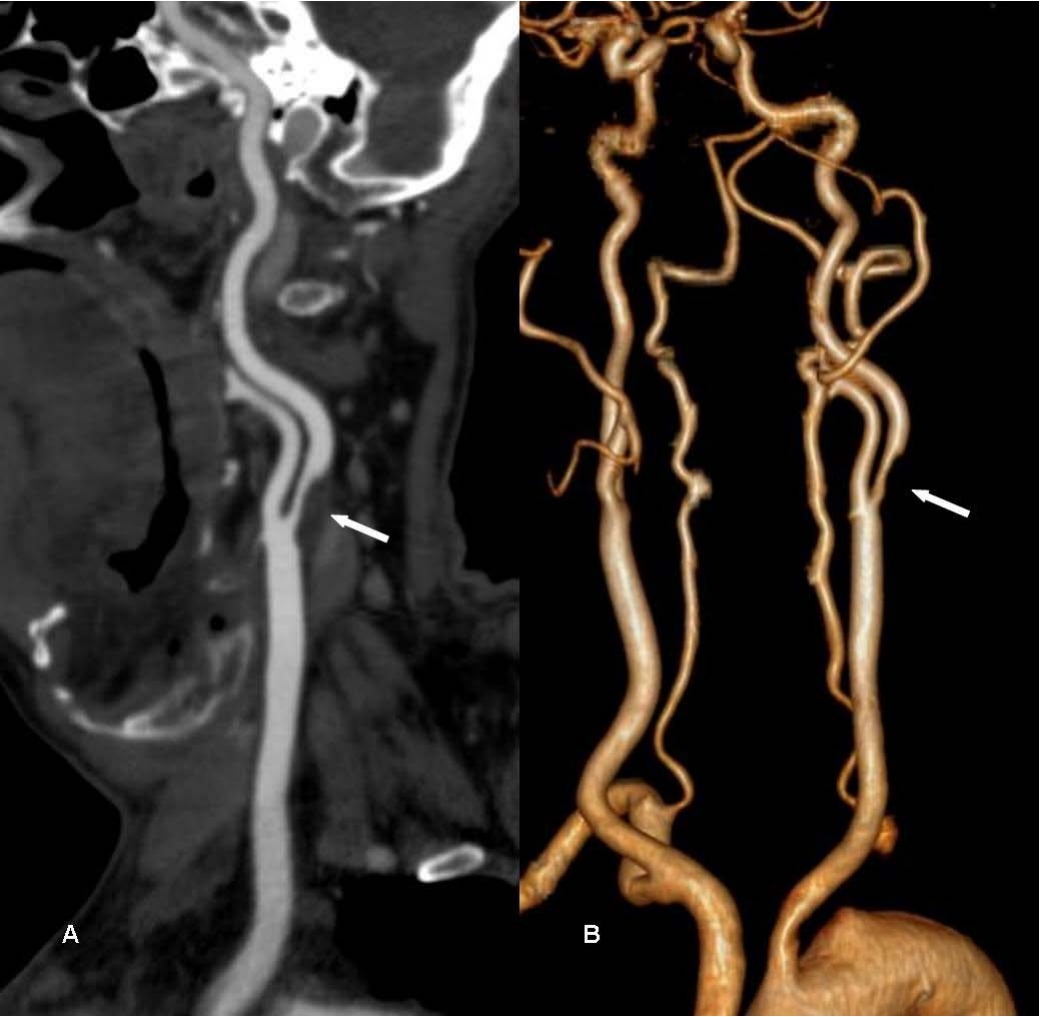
CTA images of a 51-year-old man with 13 years diabetes. A, a stenosis ≥70% in left common carotid artery proximal segment is caused by a non-calcified plaque (solid arrow) as evidenced using curved planar reformat (CPR). B, volume-rendered reconstruction (VRT) displays overview of arteries and stenosis (solid arrow).
